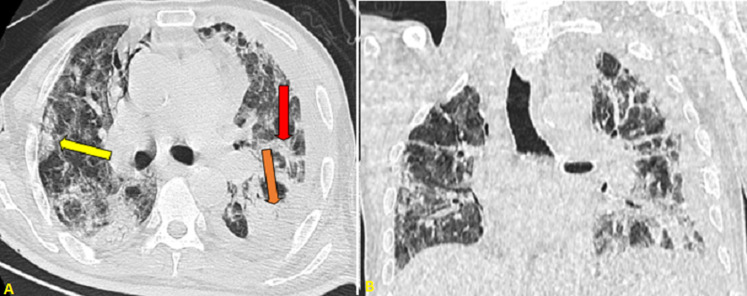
thoracic CT scan in an axial (A); and coronal (B) window objectifying ground glass opacities associated with crazy paving (yellow arrow) and fibrotic bands (red arrow), as well as consolidation in lower lobes (orange arrow)
